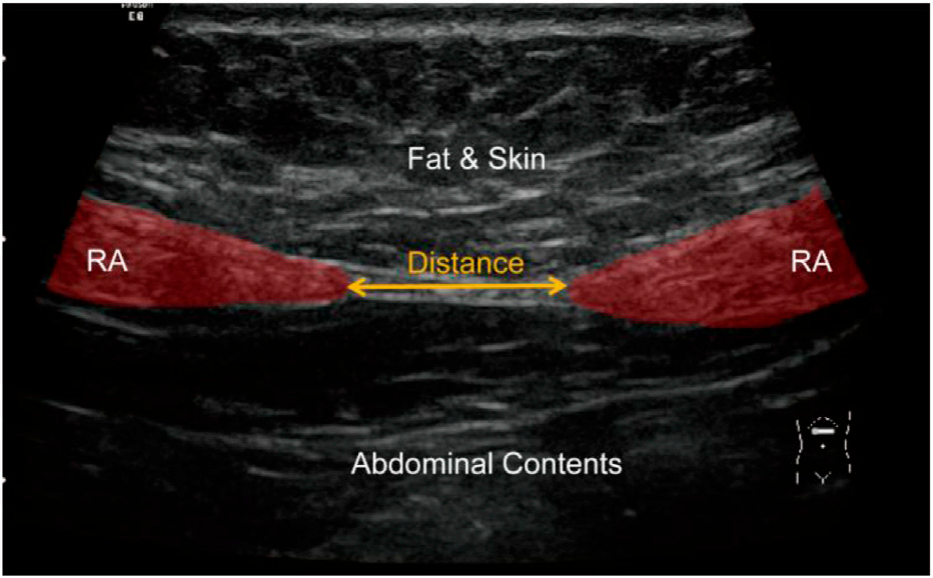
An ultrasound image showing RA (red regions) and measurement of RA distance (yellow arrow). RA, rectus abdominis.
